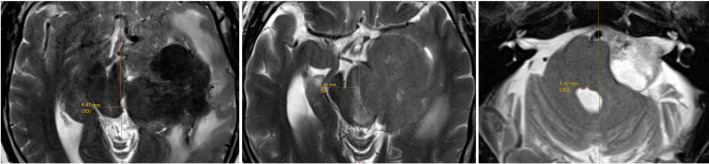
The maximal midline shift of brainstem is measured, using readily identifiable anatomical landmarks, namely cerebral aqueduct (left), mid-point of interpeduncular fossa, and median sulcus of the fourth ventricle in the T2WI MRI.
